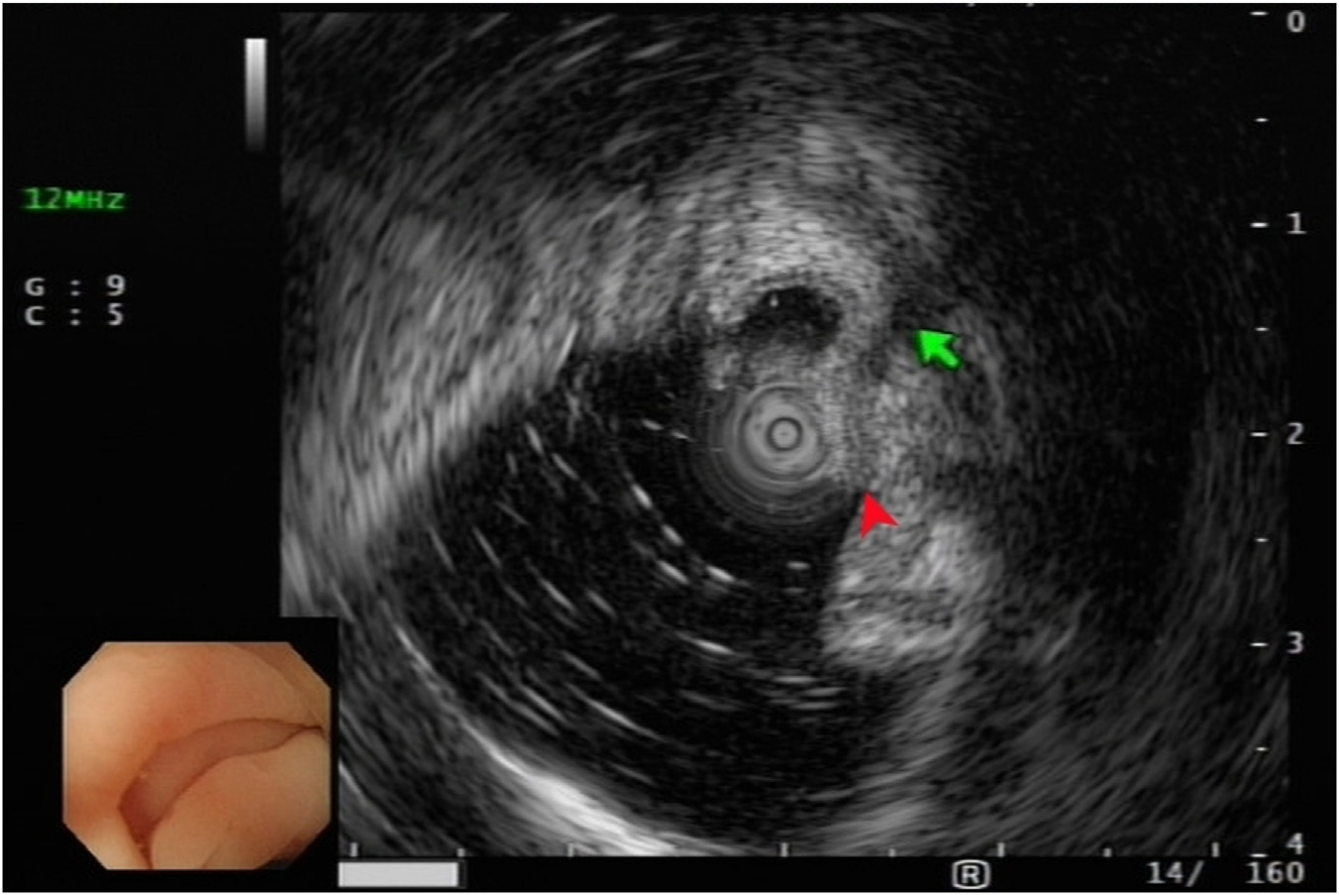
Endoscopic ultrasound image displaying appendiceal orifice (*red arrowhead*) and distended appendix (*green arrow*).
